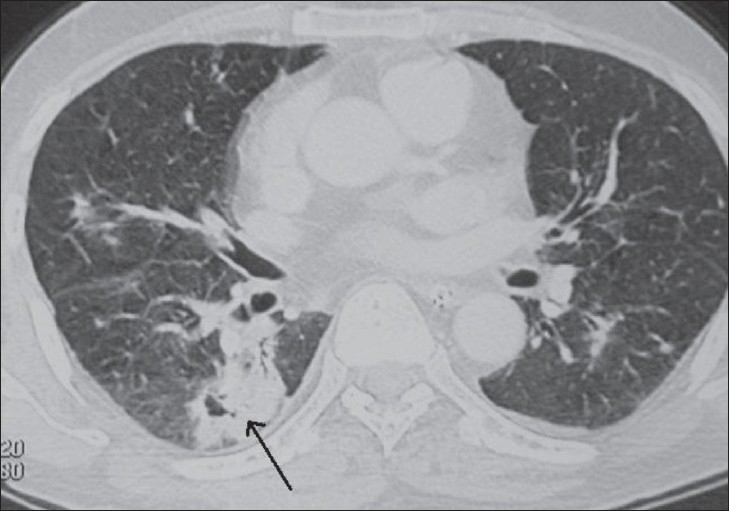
CT scan of the chest shows consolidation and collapse with internal breakdown in the superior segment of the right lower lobe (arrow)
